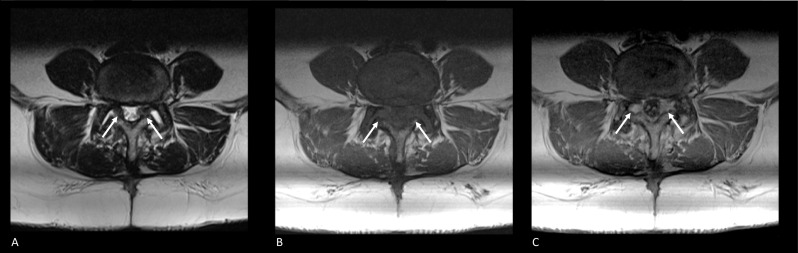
Axial MRI of the postoperative lumbar spine at level L4–L5. A Axial T2-weighted image showing soft tissue occupying the subarticular zone bilaterally. Tissue shows to have an intermediate signal intensity. Cauda equina roots are displaced and course more central in the dural sac. B Axial T1-weighted image showing the tissue characteristics of the known soft tissue in the bilateral subarticular zone. On native T1-weighted images, an intermediate signal intensity is observed. C Post-contrast axial T1-weighted image showing homogeneous enhancement of the known soft tissue in the subarticular zone bilaterally. Based on this enhancement, a suggestion of postoperative epidural fibrosis was made
